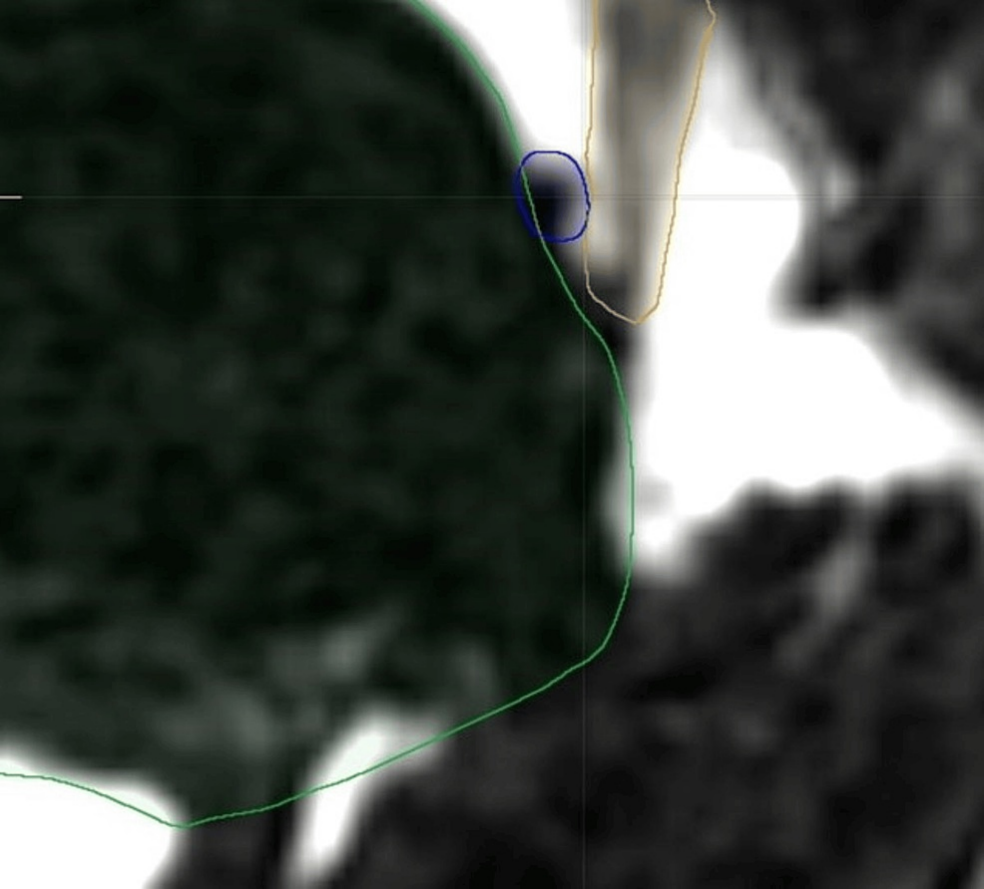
Segmentation of transverse axial MRI-CISS sequence of a 63-year-old female patient with left-sided trigeminal neuralgia. In this image, the brainstem is marked in green color, the left trigeminal nerve in brown color, and the causative venous vessel in blue color. MRI: magnetic resonance imaging; CISS: constructive interference in steady state
